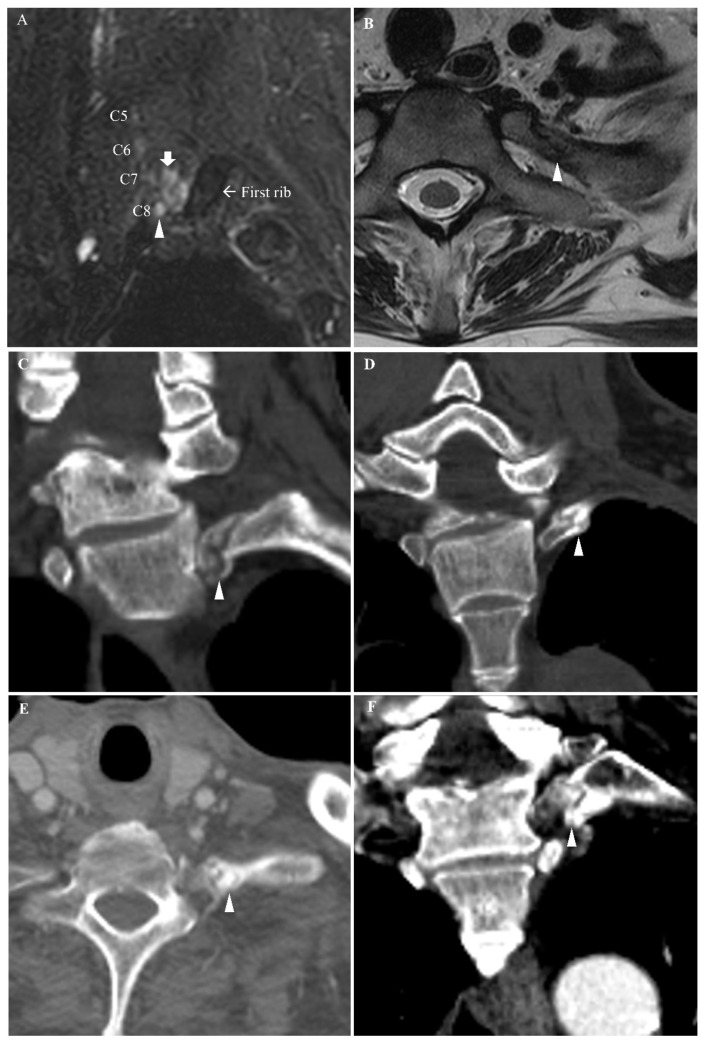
(Case 1: (A,B)) Brachial plexus and cervical spine MRI findings. (A) Parasagittal T2-weighted fat suppression image shows increased T2 signal intensity at left C8 extraforaminal nerve root (arrowhead). T2 Hyperintense area between left C8 root and the left first rib suggests soft tissue edema (short arrow). (B) Axial T2-weighted image of the cervical spine shows oblique fracture at the neck of the left first rib between the costovertebral joint and the costotransverse joint without displacement or dislocation (arrowhead). (Case 2: (C,D)) Coronal chest CT images show (C) nondisplaced fractures of the neck of the left first rib (arrowhead) and (D) the second rib (arrowhead) without dislocation at the costovertebral and costotransverse joints. (Case 3: (E,F)) Callus formation was noted at each fracture site. Chest CT images show oblique fracture of the neck of the left first rib and callus formation (arrowhead) in (E) axial image and (F) coronal image.
